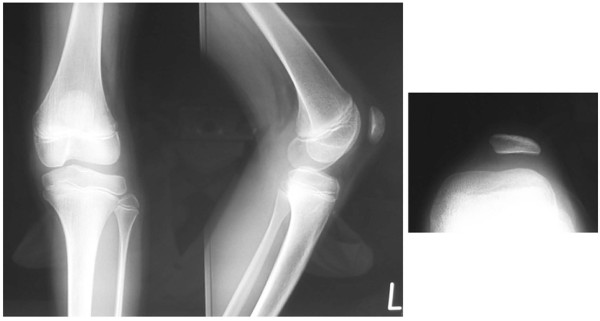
Plain X-ray taken by the referring physician 1 year after dislocation and 1 year before surgery. Although the patella locates high (Insall Salvati Ratio; 1.2), and the trochlea is shallow (sulcus angle; 168°), the X-ray confirms that the patella is in position.
